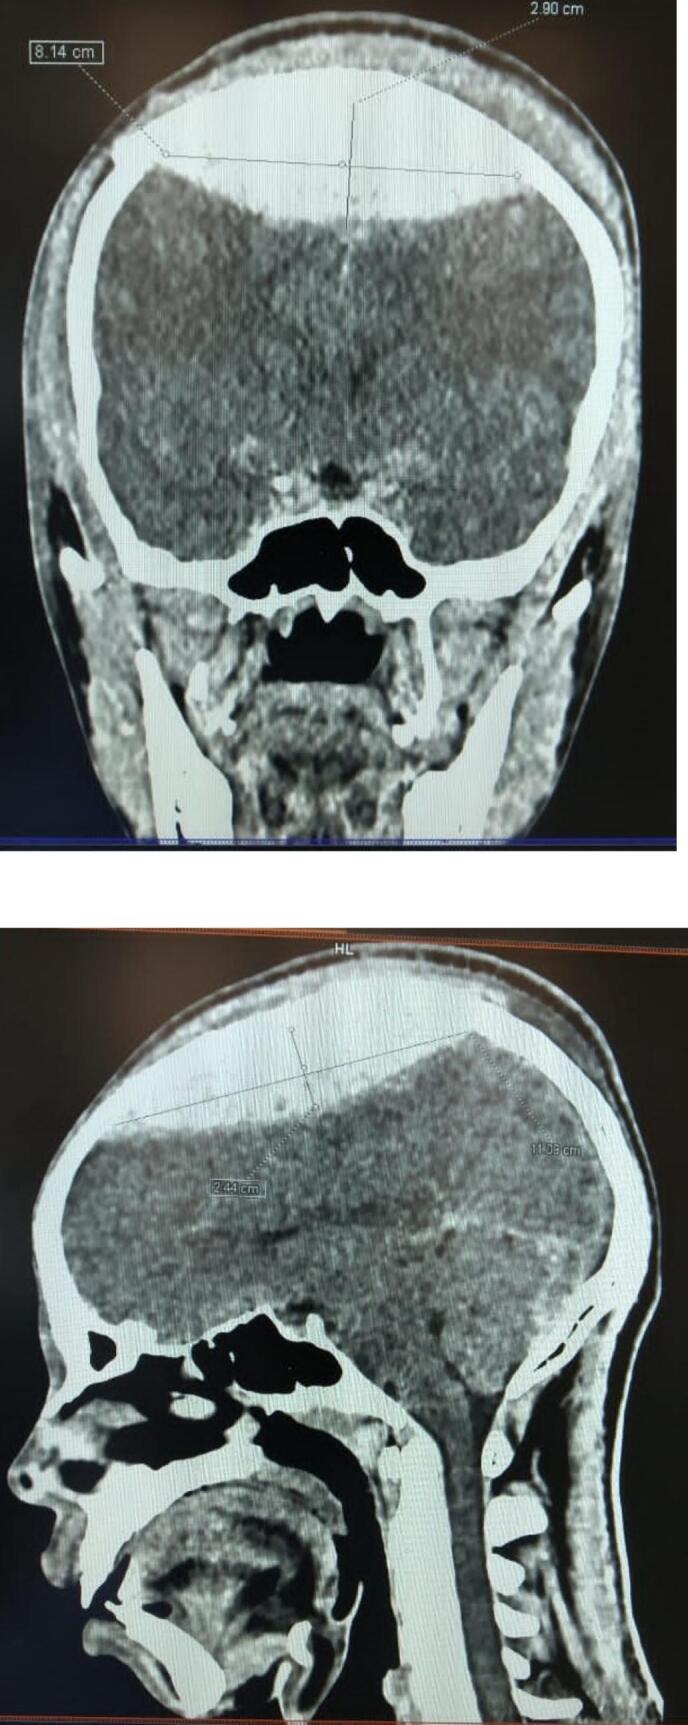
CT scan images showing large acute vertex epidural haematoma crossing the midline.
